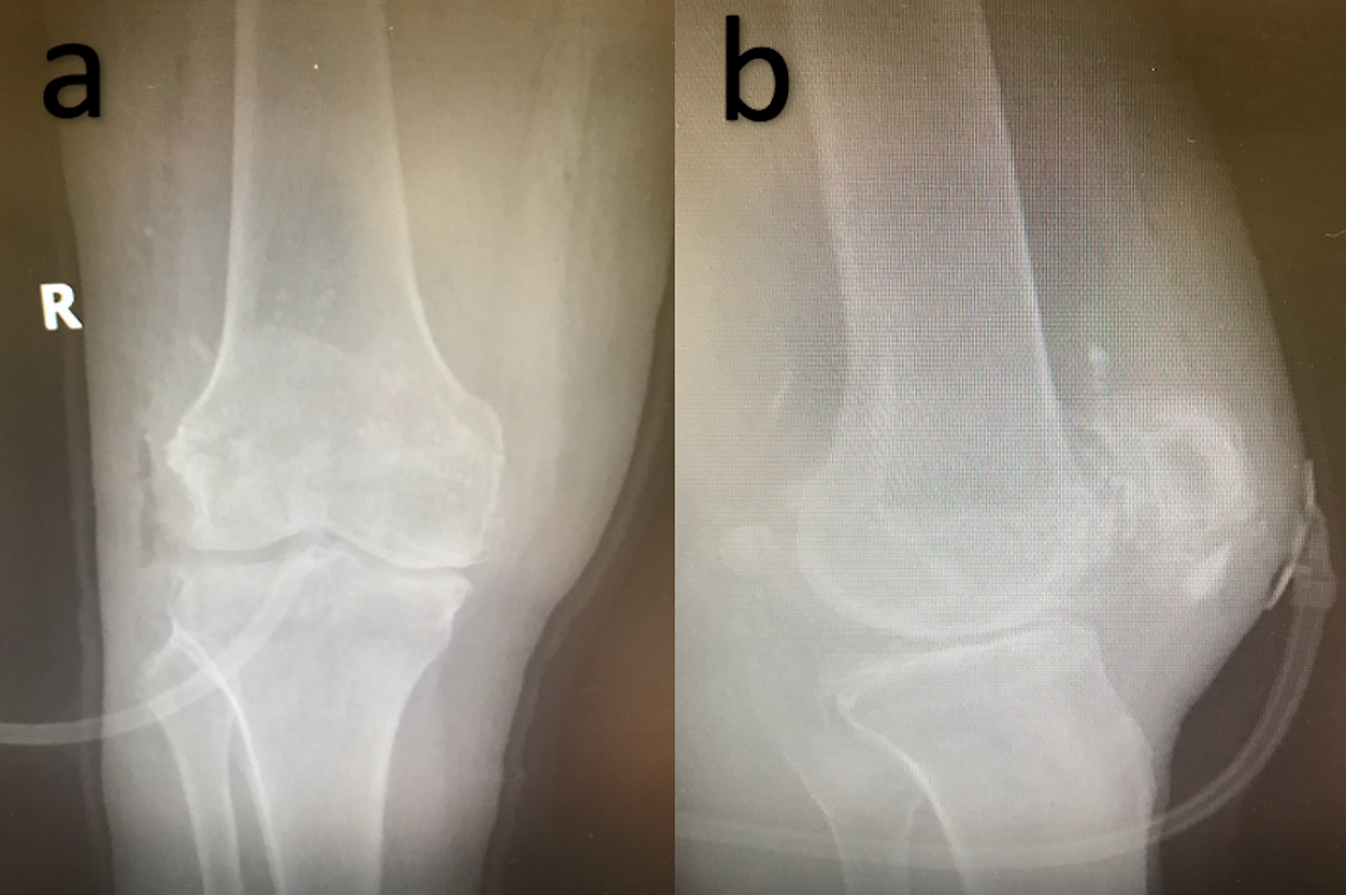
Post fracture repair X-rays Anteroposterior (AP) (a) and lateral (b) X-rays demonstrate successful repair of patellar fracture and retinaculum. Negative pressure dressing is observed in place.
